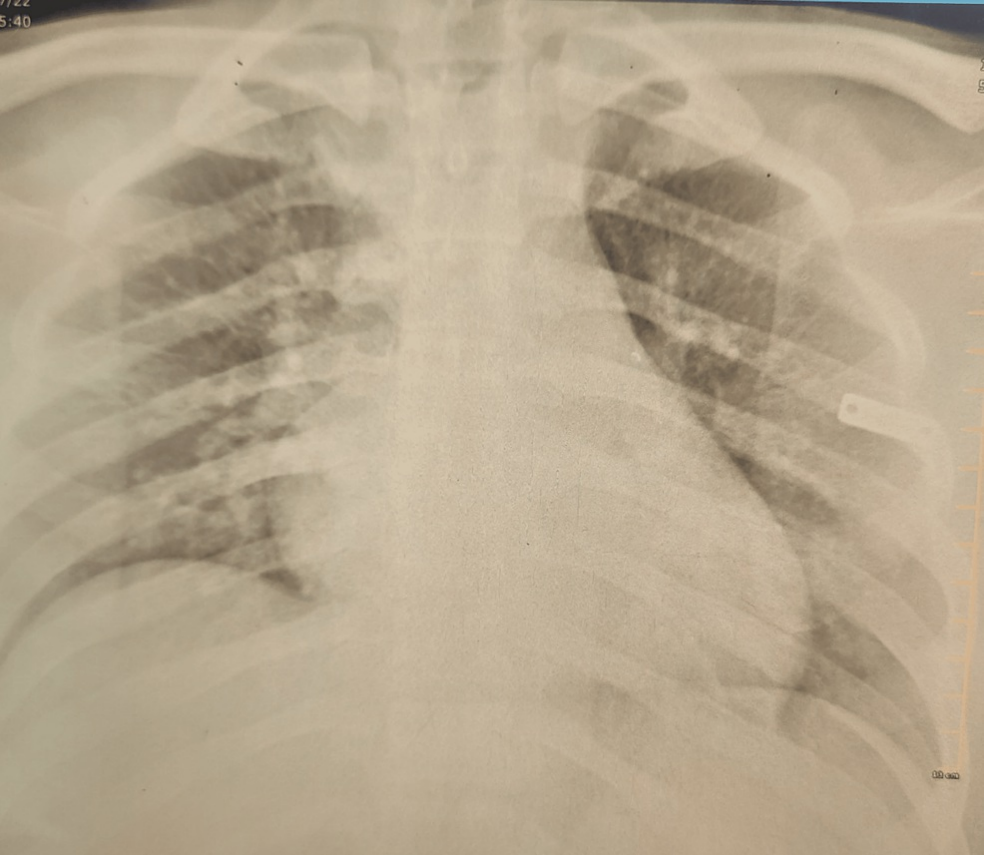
Chest X-ray anteroposterior view showing bilateral infiltrates.
